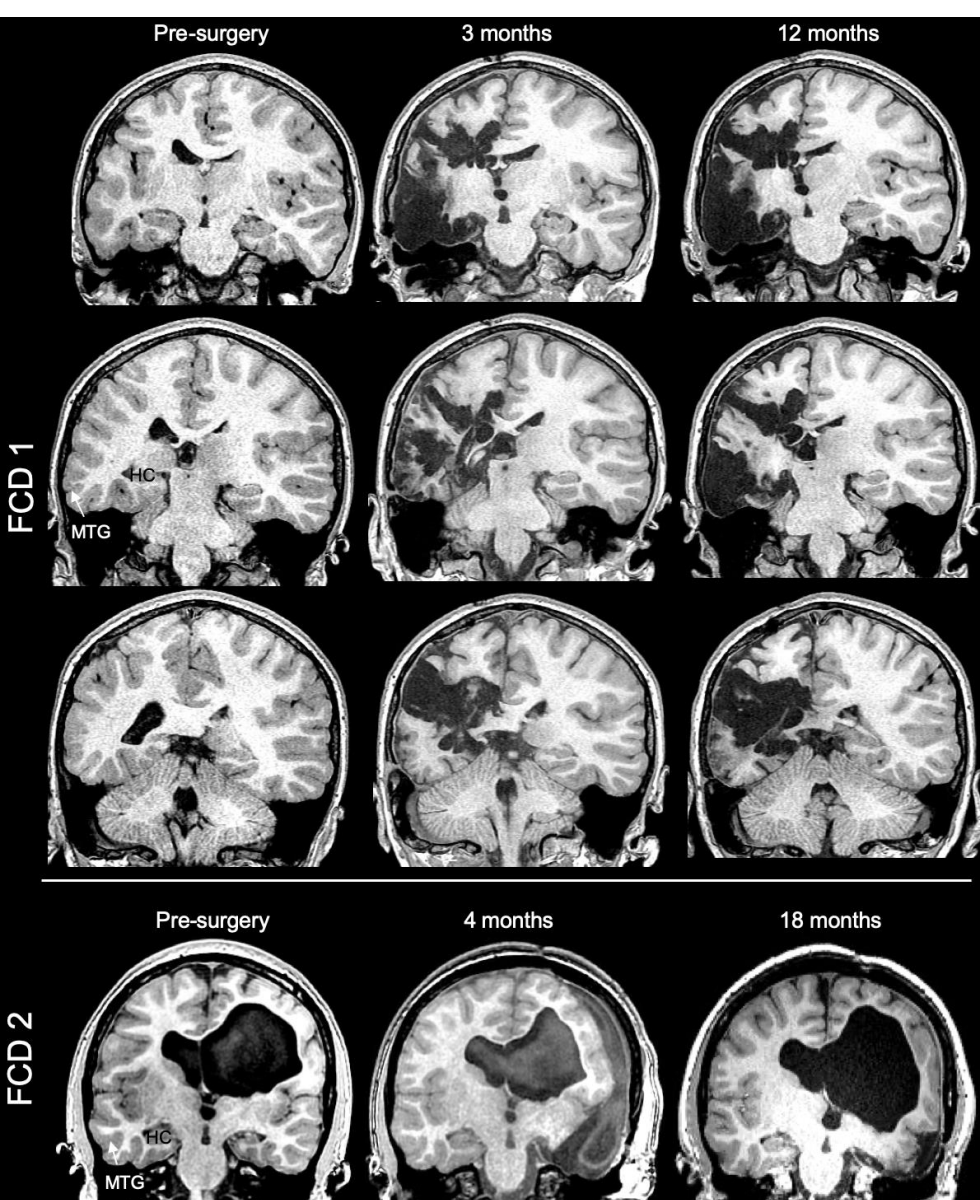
Pre- and post-surgery 3T MR imaging of both FCD cases. Extensive polymicrogyria was evident in extensive regions of the left hemisphere of FCD1, but especially the temporal lobe exhibited evidence of cortical malformation. In contrast, FCD2 experience a global injury during a premature birth that included hypointense subcortical white matter in the left hemisphere and periventricular hemorrhagic infarction in the right hemisphere that resulted in a hydrocephalus ex vacuo. Following case discussion by an interdisciplinary epilepsy surgical board, the subjects underwent extensive tissue resection of the suspected dysplastic regions driving seizure activity. FCD1 underwent a partial left hemispherectomy, whereas a right temporal lobectomy was recommended for FCD2. In both cases, surgical resection controlled seizure activity at 1 year post-surgery.
